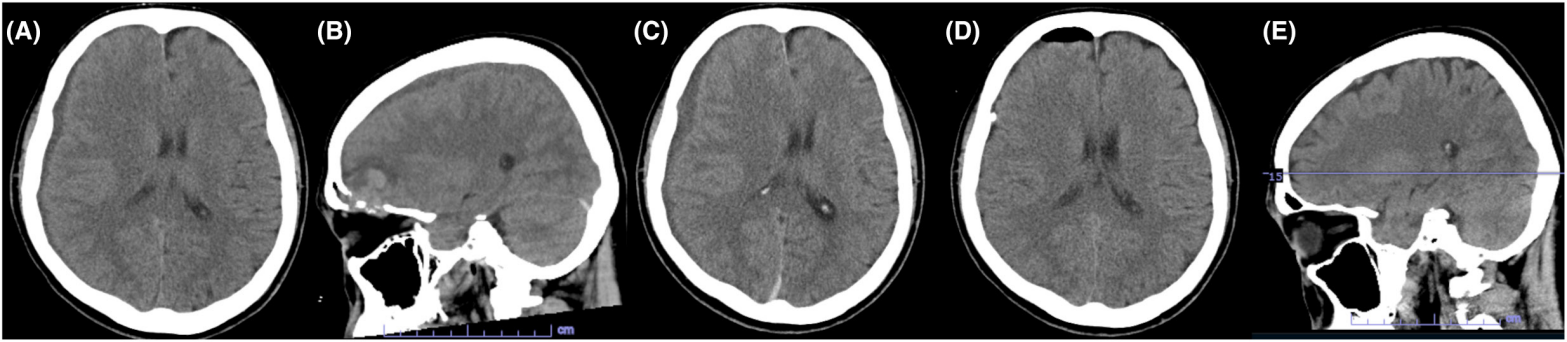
Head computed tomography (CT) scans showed a subdural hematoma communicating the skull base bone metastasis on admission (A, B). Head CT scans showed an increase in hematoma size and an exacerbation of midline shift 9 h after admission (C). Head CT scans showed reduced hematoma and improved midline shift on the second day of hospitalization (D). Head CT showed markedly reduced skull base bone metastasis 2 months after initiating Osimertinib (E).
